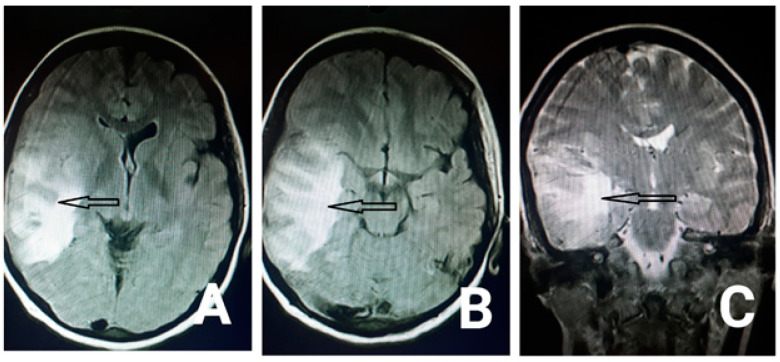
Axial fluid-attenuated inversion (flair) MRI (the arrows in the Figure A,B) and coronal T2 weighted image (the arrow in the Figure (C)) show hyperintensity in the right temporal lobe in the drainage territory of the vein of Labbe.
